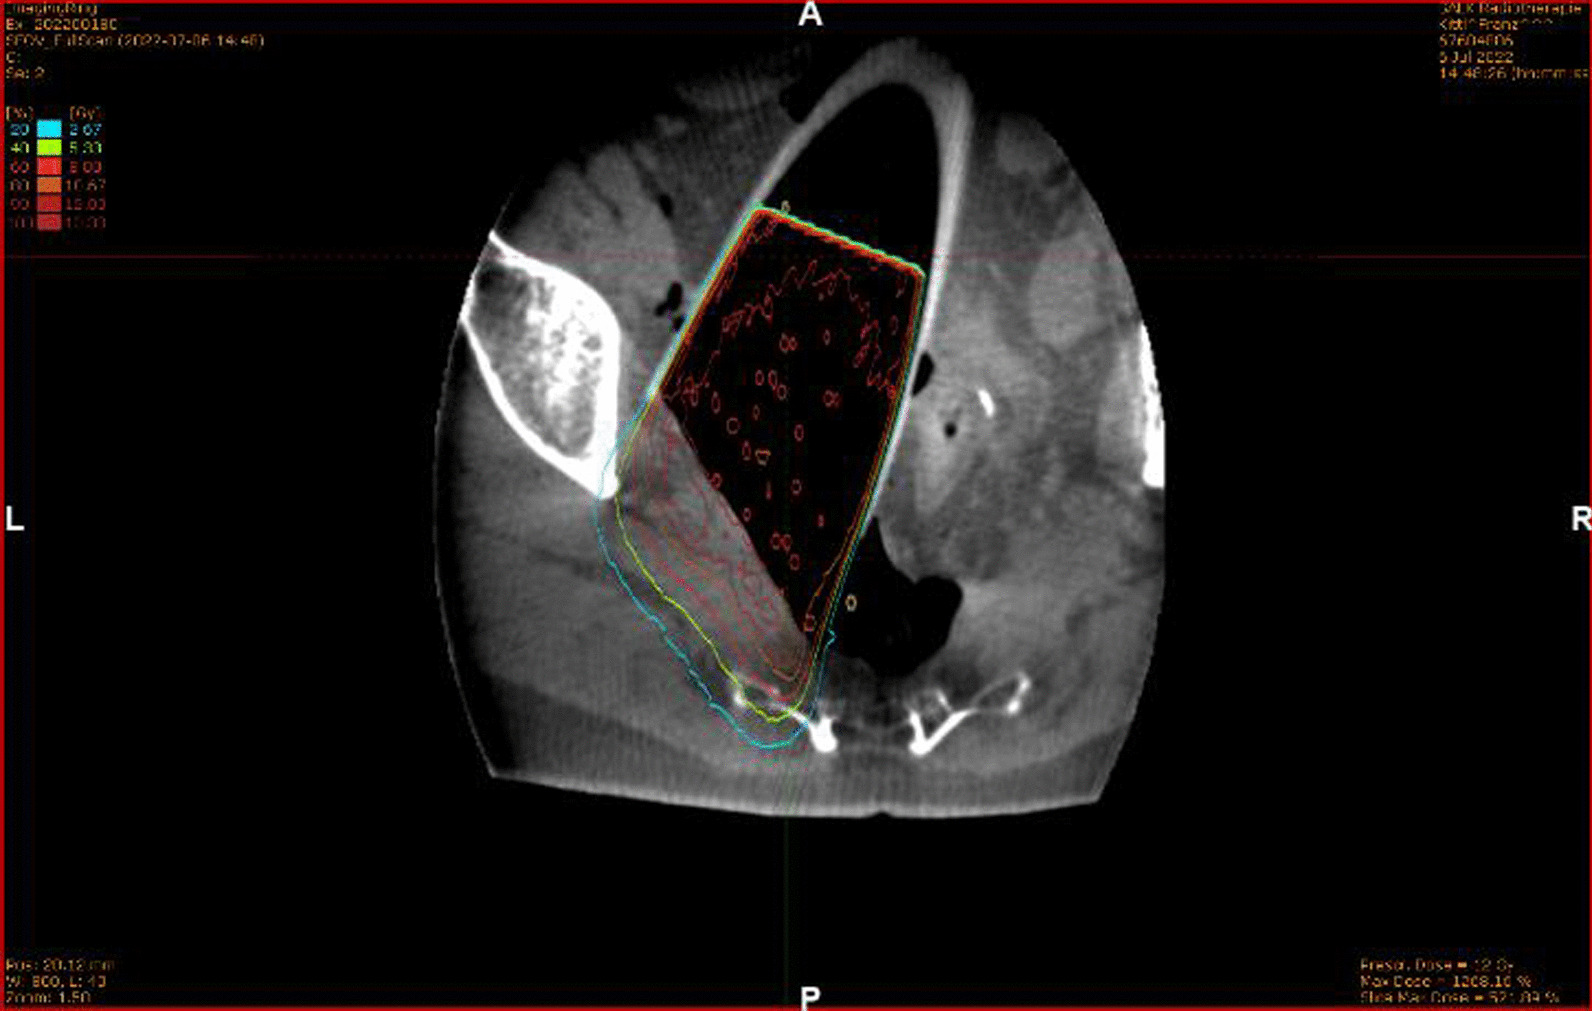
Dose distribution calculated on intraoperative cone-beam CT
